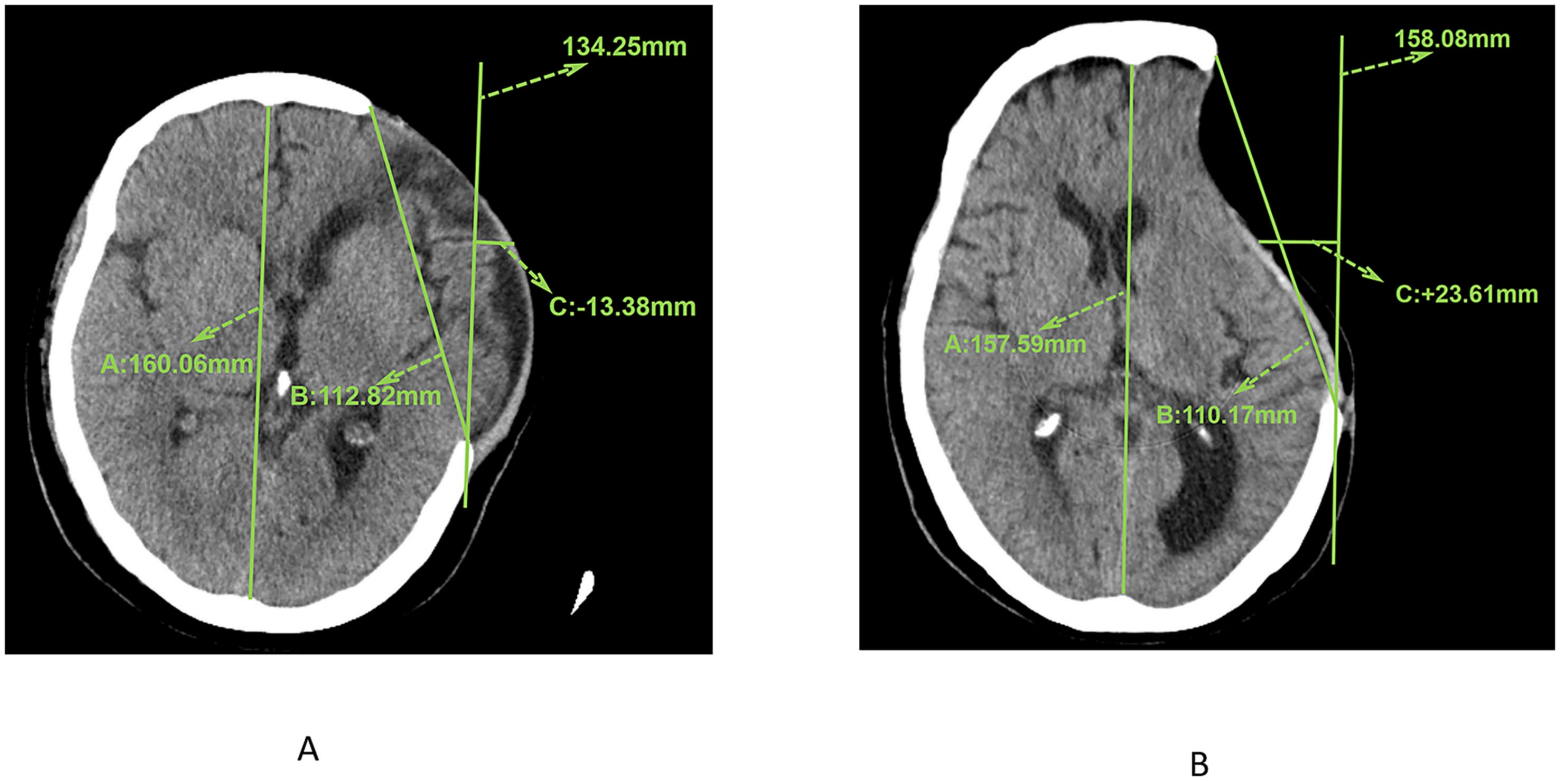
(A) CT image showing the measurement results of the maximum axial craniectomy size (110.17 mm) and skin flap shift (23.61 mm). (B) CT image showing the measurement results of the maximum axial craniectomy size (112.82 mm) and skin flap shift (13.38 mm).
